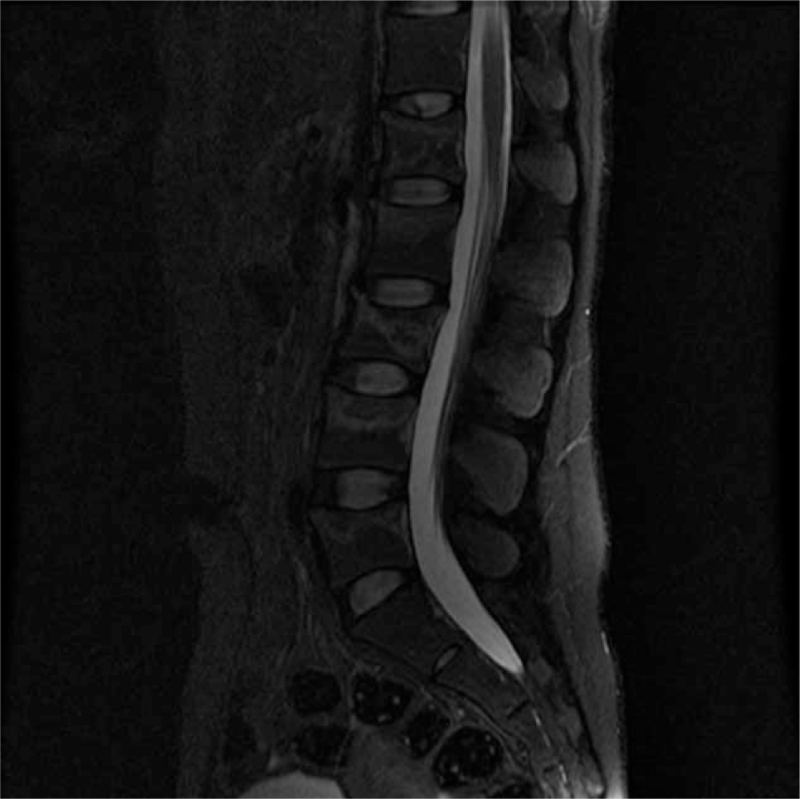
T2-weighted lumbar spinal magnetic resonance image showing trabecular compression fracture, multiple vertebrae (L1–5), intact posterior elements, no bulging disk, and no abnormal signal in cord.
